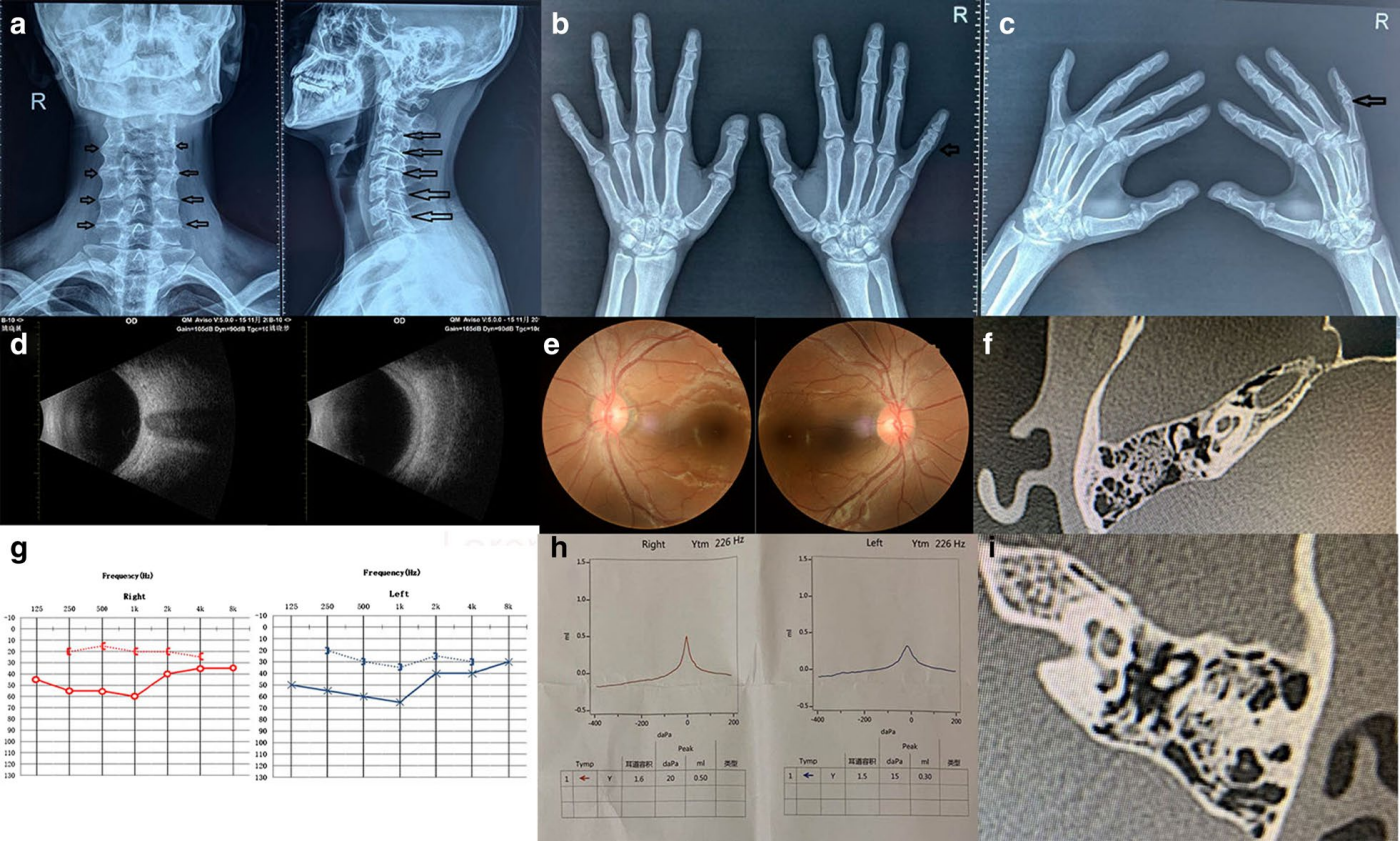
The audiological and physical examination of the probands a cervical verte bra degener ation by X-ray examination; b, c Joint space ambiguity by X-ray exam ination; d Thickened bulb in both eyes; The vitreous body of both eyes is punctate turbid by ultrasonography, e no obvious abnormality by fundus camera; g Binaural conductive hearing by PTA; h "A" type by acoustic immittance measurem ent; f, i The temporal bone CT
